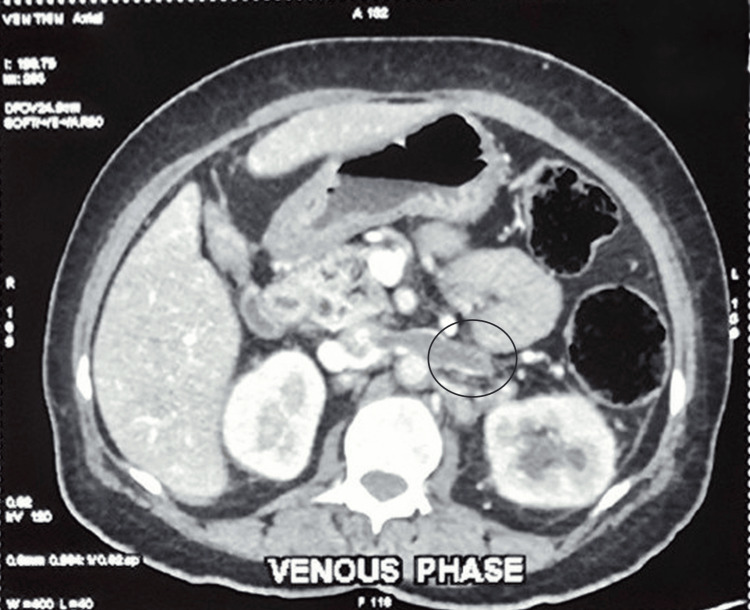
CECT venous phase showing left RVT CECT: Contrast-enhanced CT; RVT: Renal vein thrombosis
